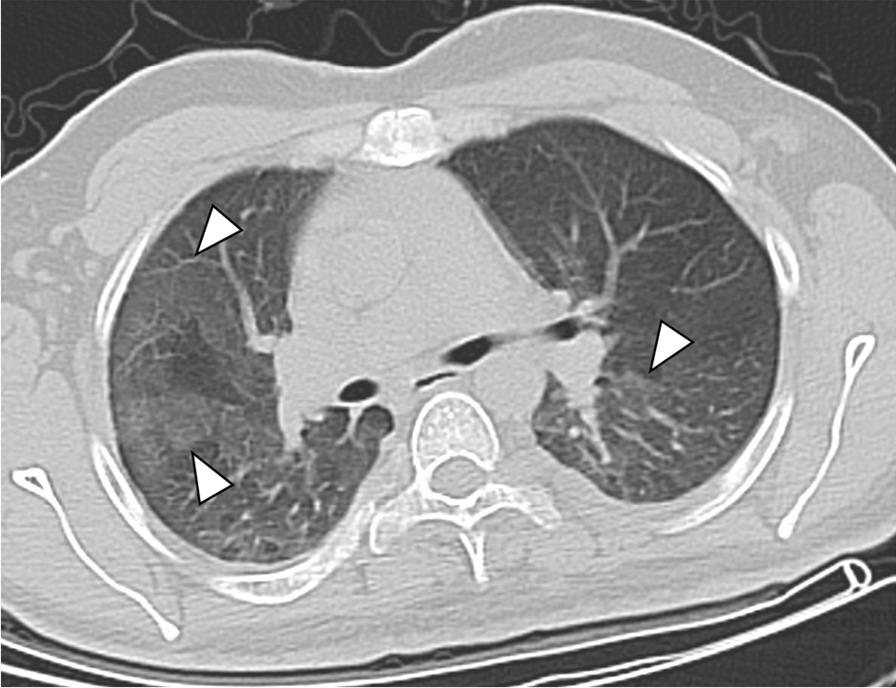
Transversal CT image of a 27-year old male non-smoker with predominantly right-sided ground glass opacifications (white arrowheads)
